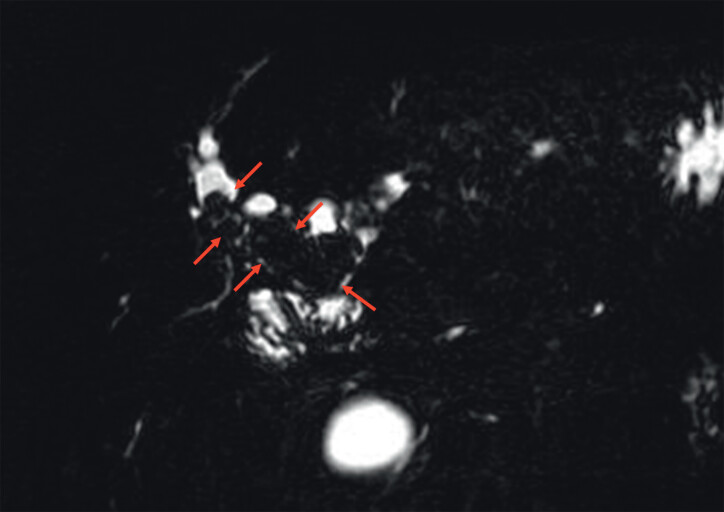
Magnetic resonance imaging revealing multiple large stones in the right intrahepatic bile duct (red arrow).
